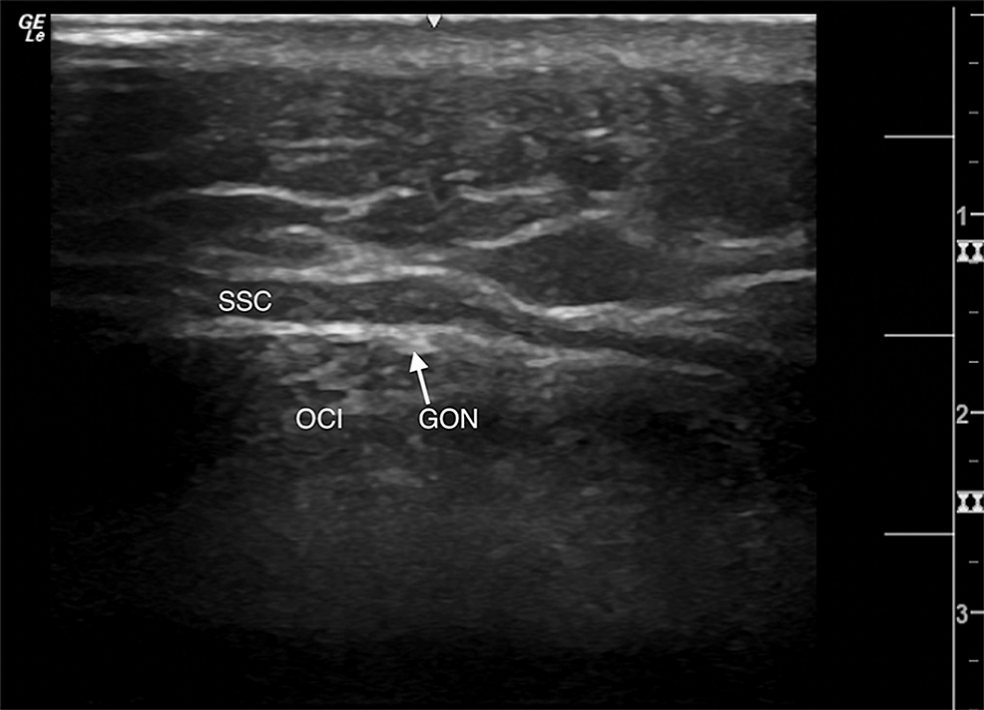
Left-sided greater occipital nerve (GON) seen with high-frequency linear ultrasound probe SSC: semispinalis capitis; OCI: obliquus capitis inferior.
